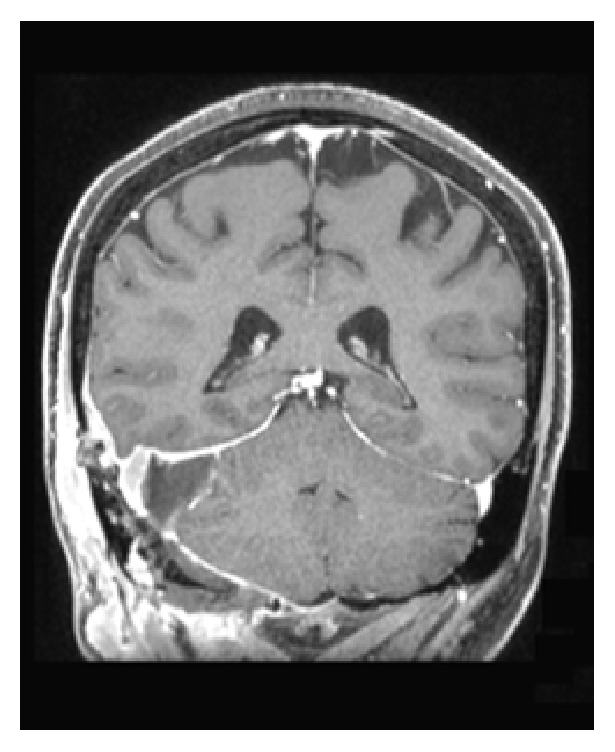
Coronal view of contrast-enhanced MRI scan following resection.
